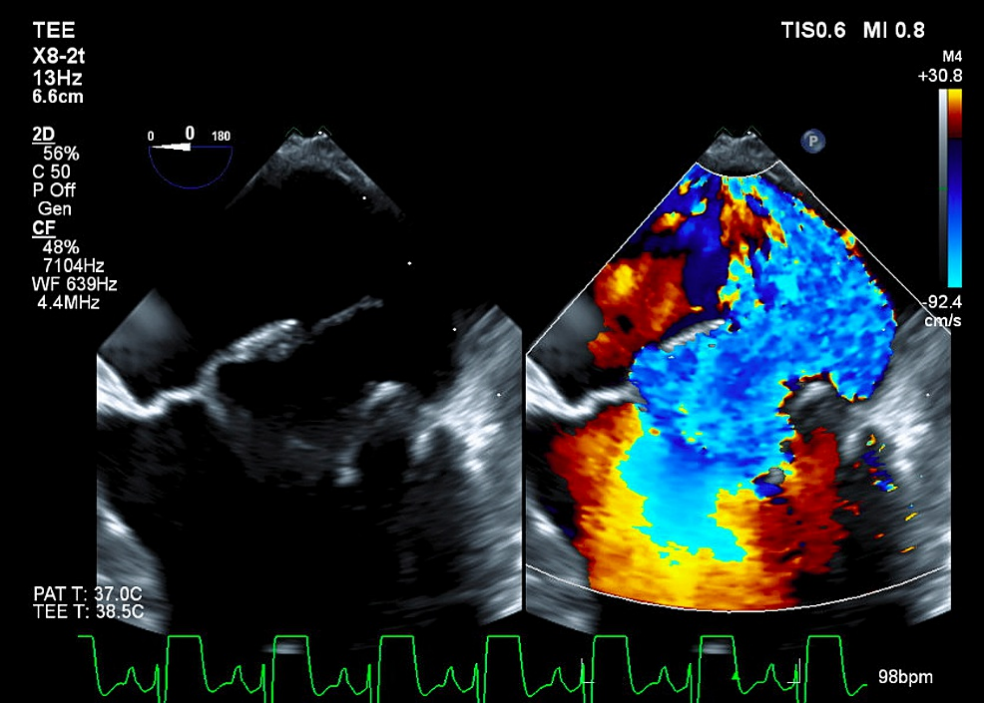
Transesophageal echocardiogram still image to better visualize severe mitral regurgitation
